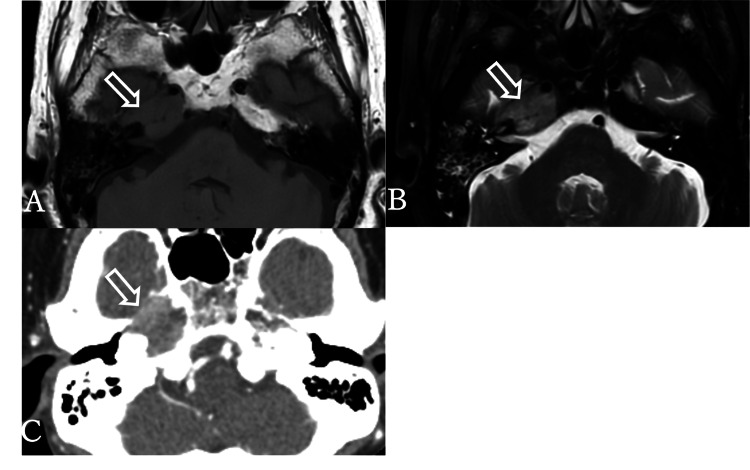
Axial MRI and CT of the temporal bone. (A) Axial T1-weighted MRI image. (B) Axial T2-weighted MRI image. A region in the right petrous apex appears isointense to brain parenchyma on T1-weighted imaging and iso- to mildly hyperintense on T2-weighted imaging. Additionally, an area in the ipsilateral mastoid, likely reflecting inflammation, is observed. (C) Contrast-enhanced CT scan performed for systemic evaluation. A tumorous lesion corresponding to the right petrous apex is identified, showing partial contrast enhancement. The lesion extends with bone destruction of the petrous bone.
